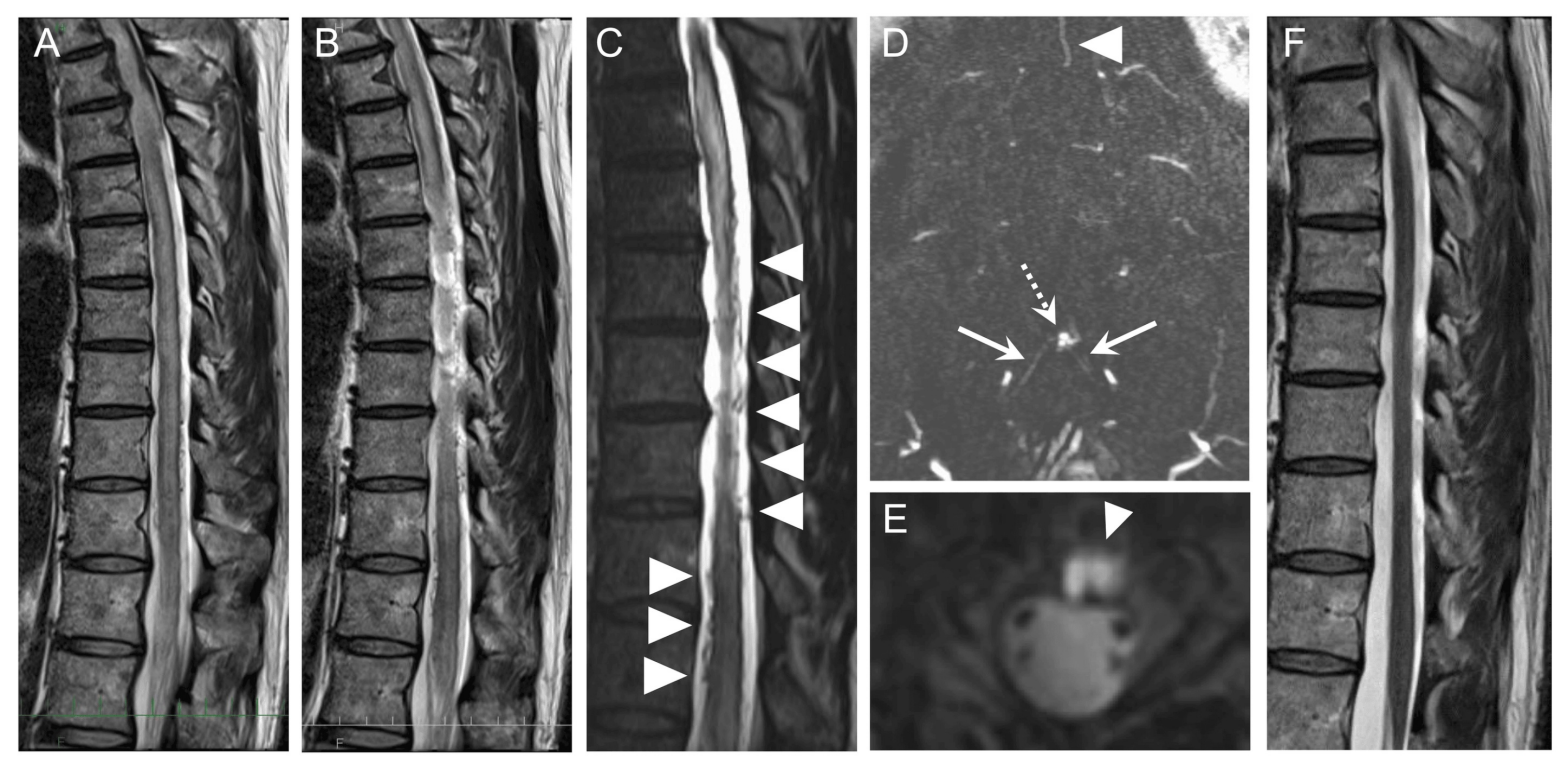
MRI findings in spinal arteriovenous fistula Sagittal T2-weighted magnetic resonance imaging (MRI) of the thoracic and lumbar spine showed diffuse hyperintensity from the upper thoracic cord to the conus, consistent with edema (A, B). Heavily T2-weighted images (heavily T2WI) demonstrated abnormal vascular flow voids in the ventral aspect of the thecal sac, suggesting a dural arteriovenous fistula (arrowheads) (C). Dynamic contrast-enhanced MR angiography (DCE-MRA) showed the shunted pouch (dotted arrow), feeding artery (solid arrows), and draining vein (arrowheads) in the coronal plane (D). A fusion image combining the reconstructed axial DCE-MRA and heavily T2WI demonstrated the presence of a venous pouch within the extradural space (arrowhead) (E). Four months after the treatment, MRI showed resolution of the abnormal signal intensity within the cord (F).
